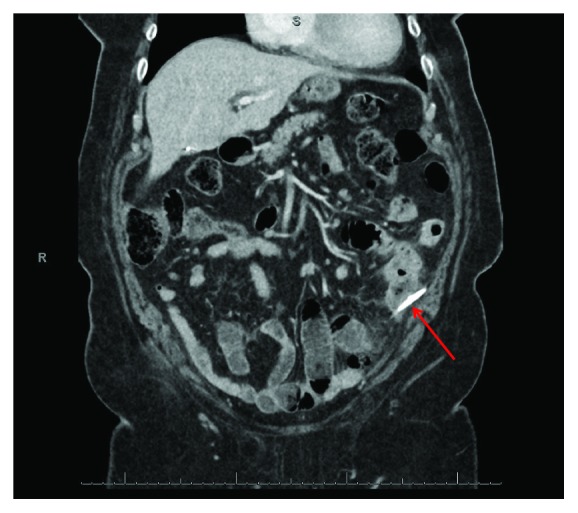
CT scan of biliary stent perforating sigmoid colon.
